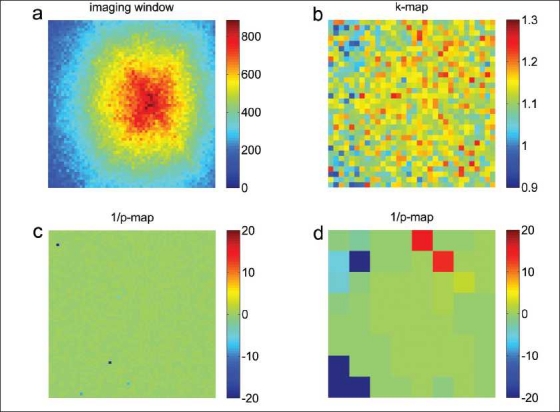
Projection data acquired by placing a 6-mm lead-shielded I-123 source in the SPECT scanner: (a) for energy window 1 (143–175 keV) in a 64 × 64 matrix size, data for energy window 2 (184–216 keV) looks similar to (a) (not shown); (b) the ratio (k map) of the two energy windows in a 32 × 32 matrix size; (c) 1/P maps in a 64 × 64 (c) and (d) 8 × 8 matrix size. The P-values correspond to Bonferroni-corrected double-sided significance levels. A 1/P value of 20 corresponds to a significance level of 5%. Negative P-values indicate negative differences.
